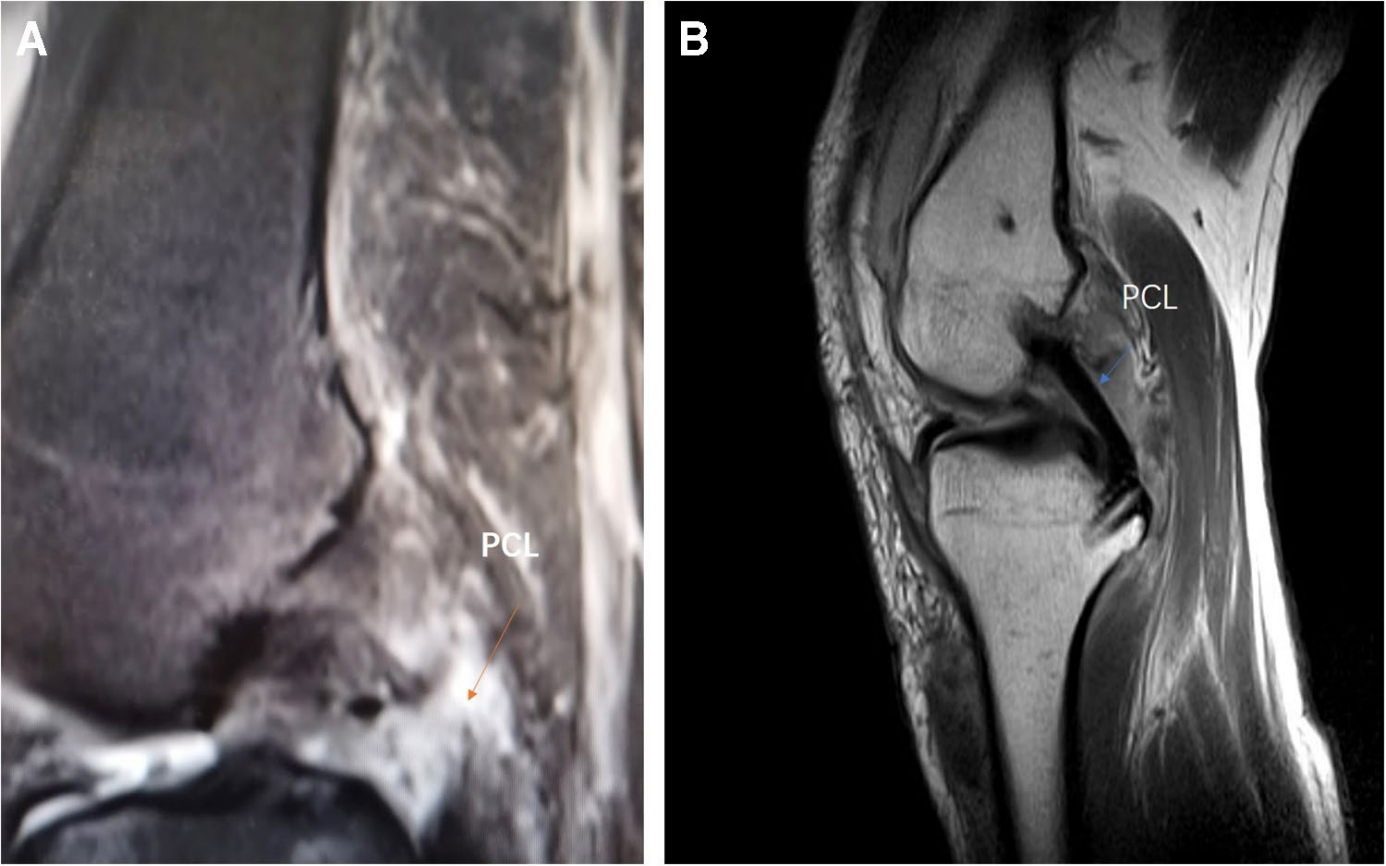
The appearance of the PCL reconstruction on magnetic resonance imaging (MRI) is shown. A Pre-operatively, a complete PCL injury was visible on MRI. B The reconstructed PCL is shown on post-operative 12-month MRI
